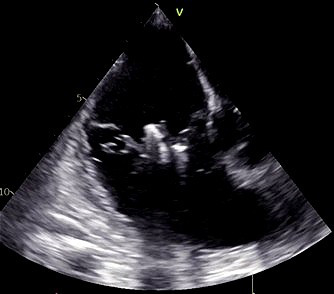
Imaging artifacts obscuring coaptation zone	Reverberation from annular calcification or device shadowing	Switch to alternate TEE planes; use 3D or intracardiac echocardiography (ICE) as needed; reduce gain and optimize depth/focus settings
